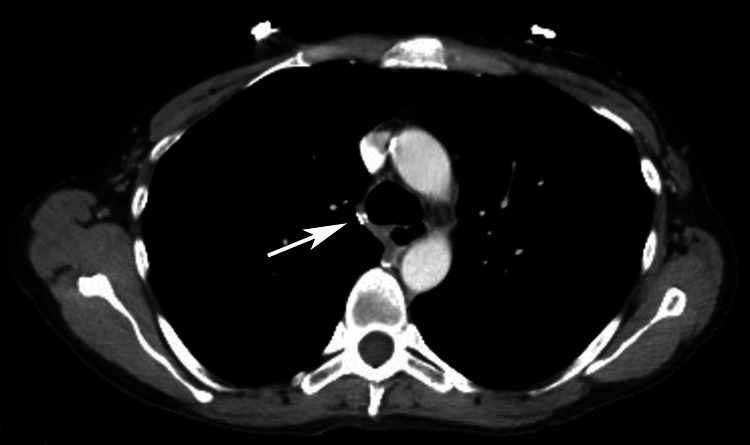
Follow-up CT of the chest with IV contrast eight months post injury showing no evidence of pseudoaneurysm.
